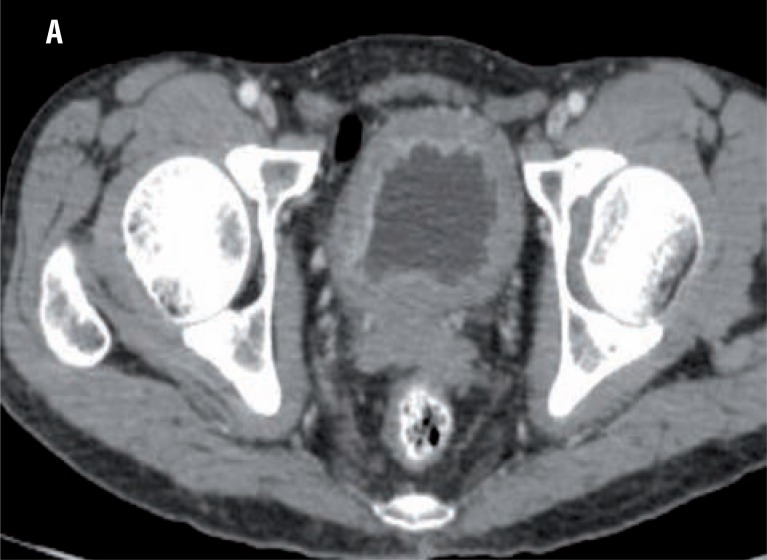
CT showing enhancing soft tissue lesion in the urinary bladder suggesting neoplastic lesion.
